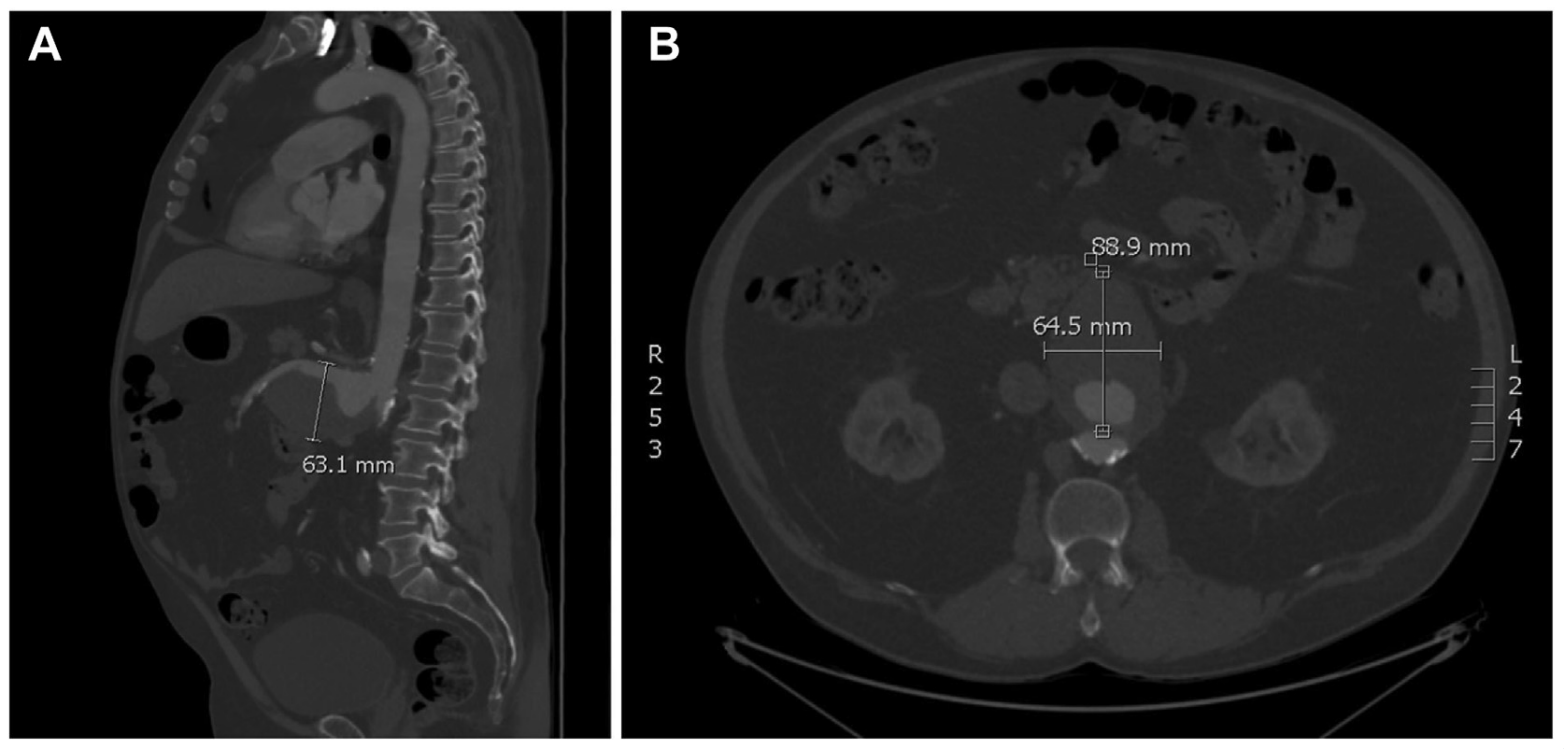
Preoperative sagittal (A) and transverse (B) computed tomography angiography images of a superior mesenteric artery (SMA) aneurysm (SMAA) measuring 9.6 cm anteroposteriorly, 6.5 cm craniocaudally, and 6.4 cm transversely in the maximal dimensions.
